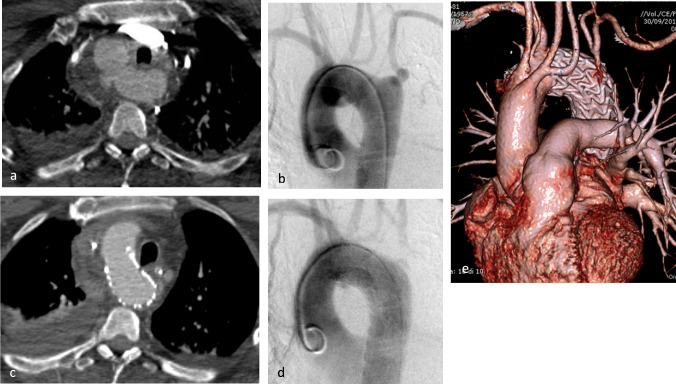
Grade III aortic isthmic lesion in Right-sided aortic arch anatomic variant treated with Gore C-TAG 31 × 150 mm. a Preoperative CTA and b aortography; c postoperative CTA and d aortography and f volume rendering: correct positioning and patency of endoprostheses, no complications
